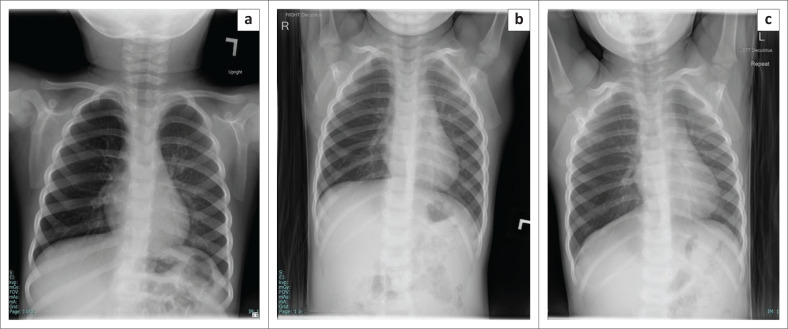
Images of a 30-month-old child with a history of almond aspiration. Although the foreign body was not radiographically visible, the chest radiograph (a) showed a hyperinflated right lung, and right lateral decubitus inspiratory (b) and expiratory films (c) demonstrated air trapping, suggesting foreign body aspiration into the right main bronchus.
